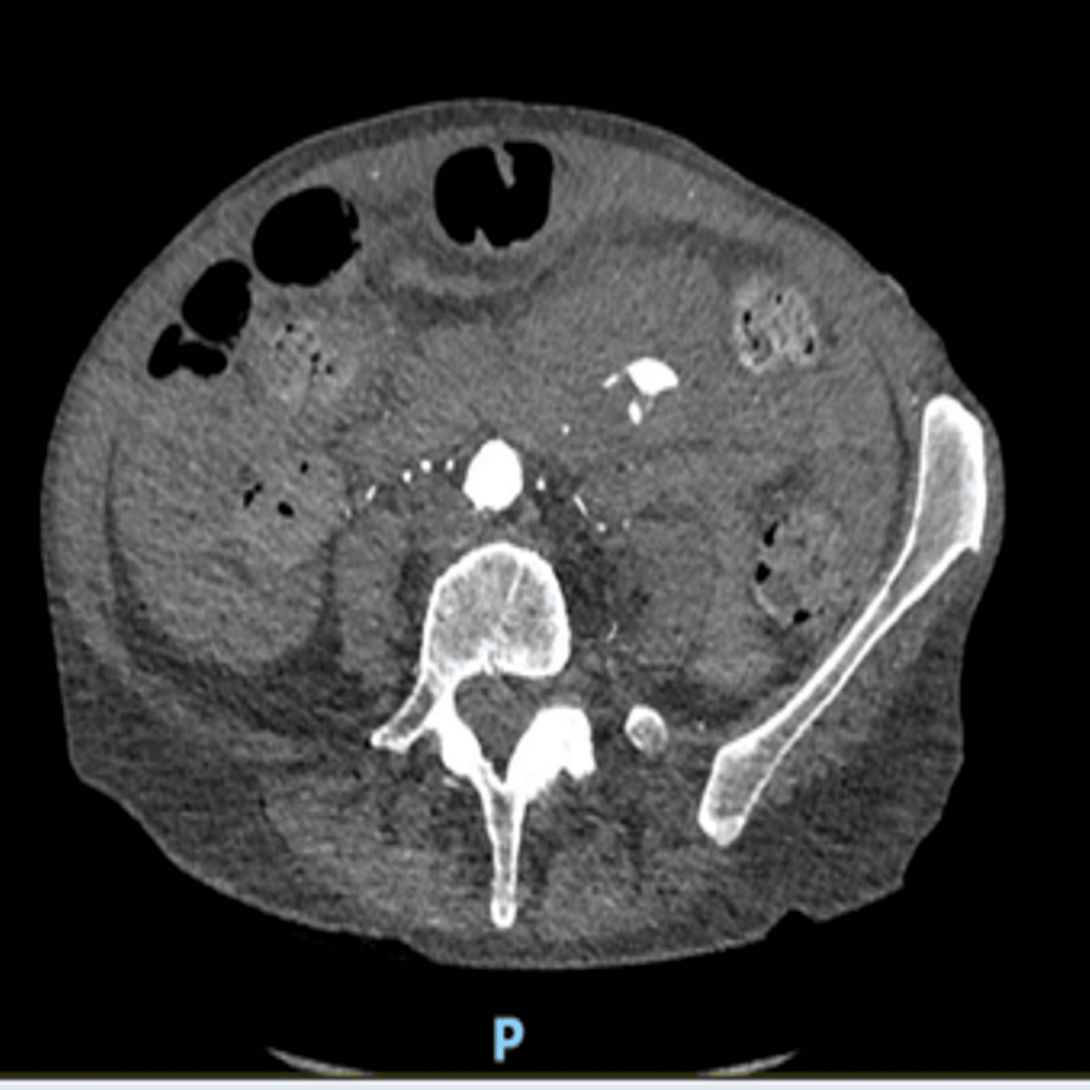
Arterial phase CT - axial view showing active blush CT: computed tomography
